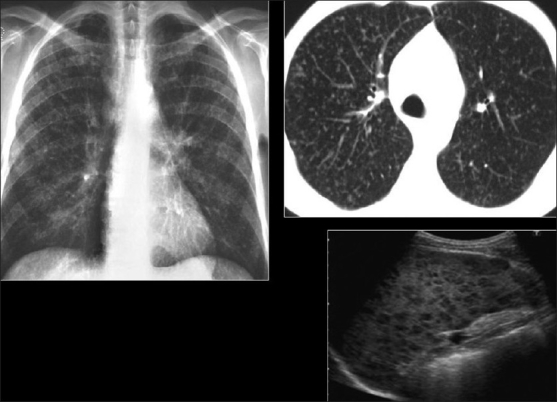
“Primary” *Mycobacterium tuberculosis (MTB)*. Chest X-ray and high-resolution computed tomography show diffuse randomly distributed miliary nodules. Ultrasound of the spleen of the same patient shows splenomegaly and multiple hypoechoic nodules due to MTB granulomas
